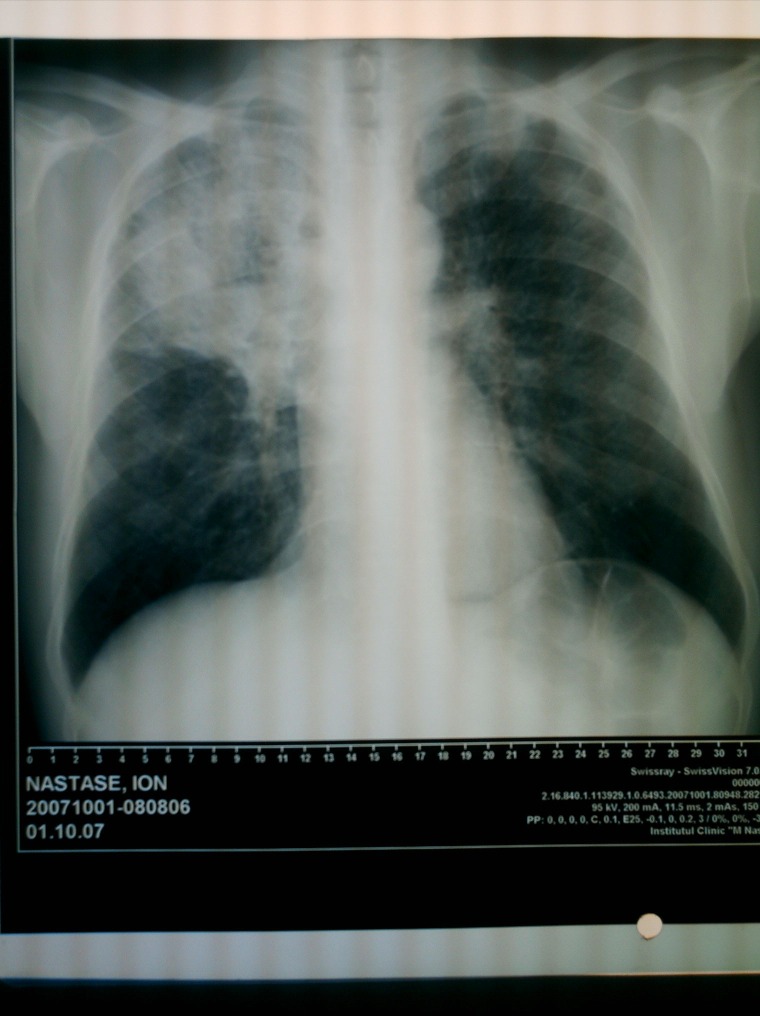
Preoperative X-ray exam - lung opacity at upper right lobe
